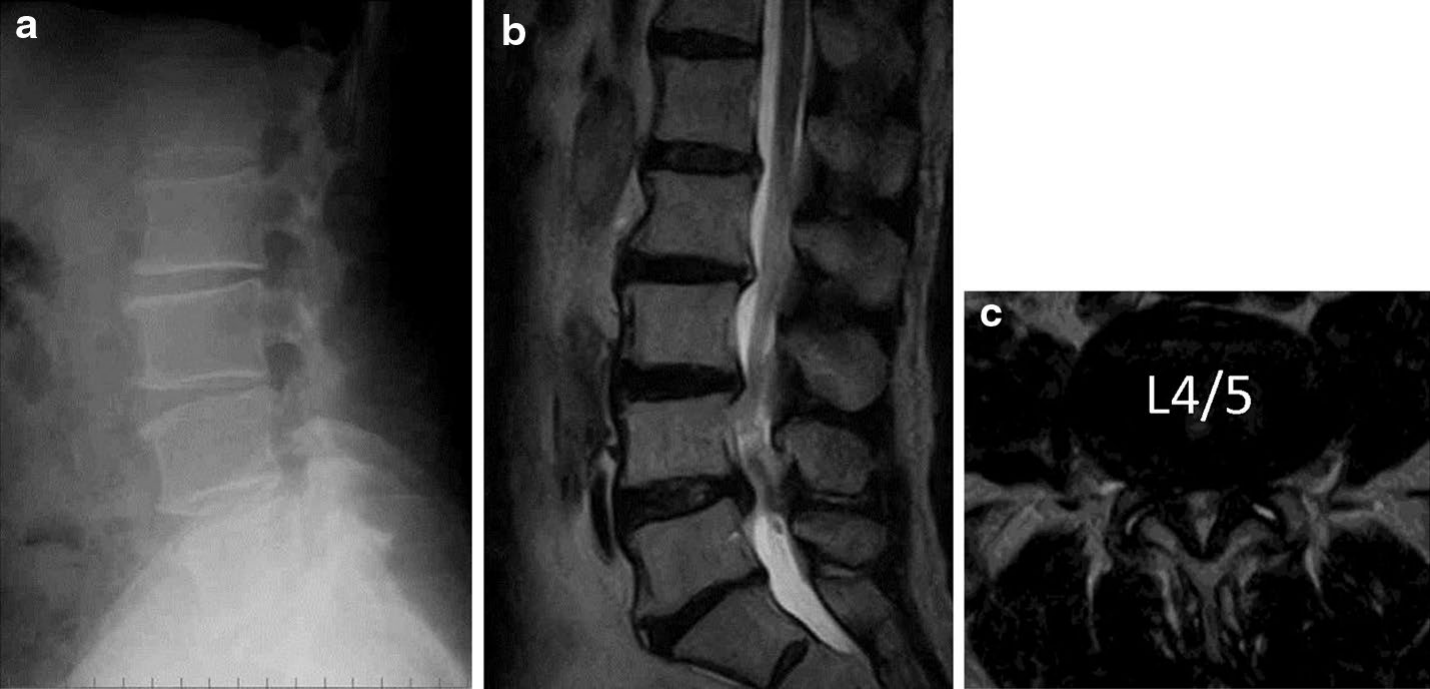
Radiographic findings of the lumbar spine. A plain radiogram of lumbar spine (a) showed slight anterior listhesis of the 4th lumbar spine. A magnetic resonance imaging showed spinal canal stenosis between 4th and 5th lumbar spinal level and redundant nerve roots superior to the most stenotic level (b, c).
